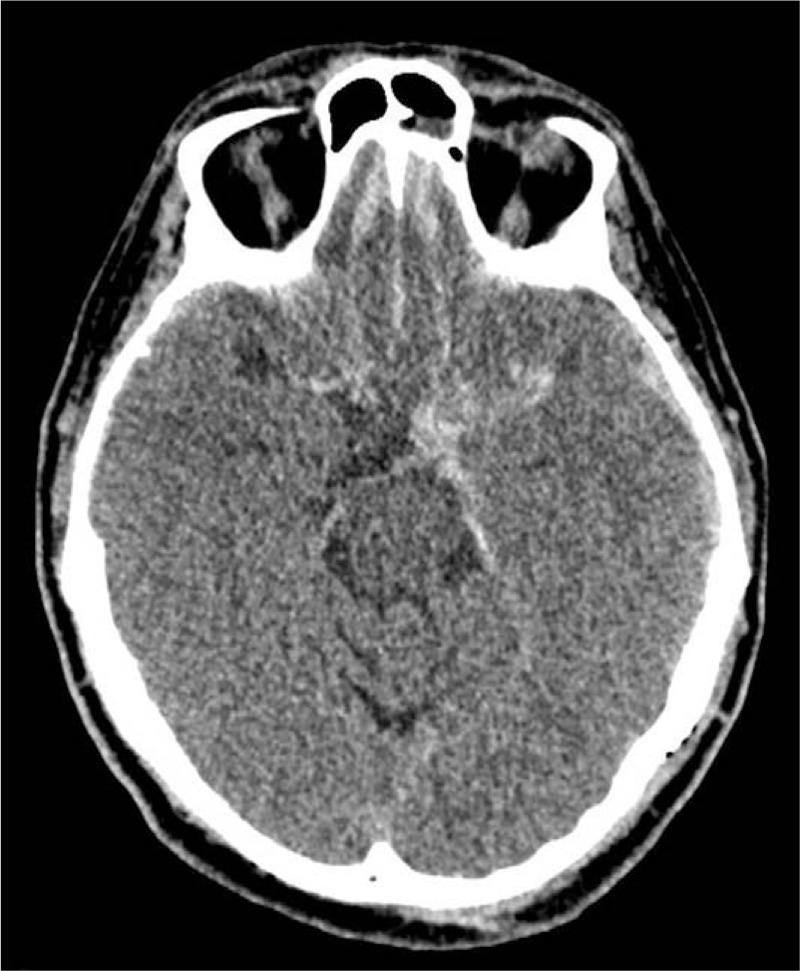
Cranial CT-scan (axial slice) showing SAH occupying the basal cisterns and left Sylvian fissure.
